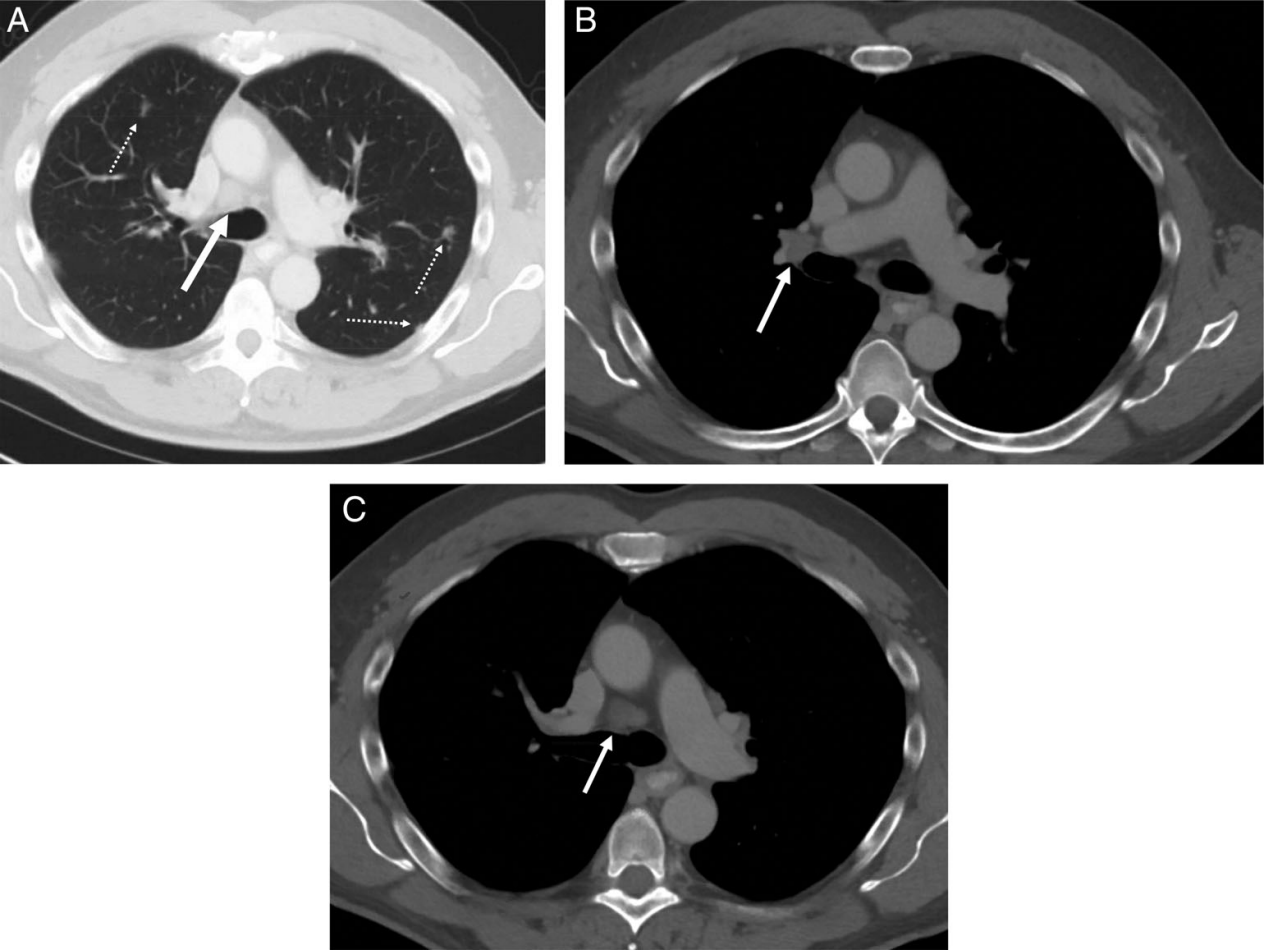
(A) A computed tomography (CT) scan of the patient's chest in lung window. Note the white dashed arrows point to pulmonary nodules found on this patient. The large white arrow shows the right pre‐tracheal lymphadenopathy (LAD). (B) A CT scan of the patient's chest in the mediastinal window, showing the right hilar LAD that measures 10.88 mm × 16.55 mm. (C) A CT scan of the patient's chest in the mediastinal window, showing the right pre‐tracheal LAD that measures 19.01 mm × 15.35 mm.
